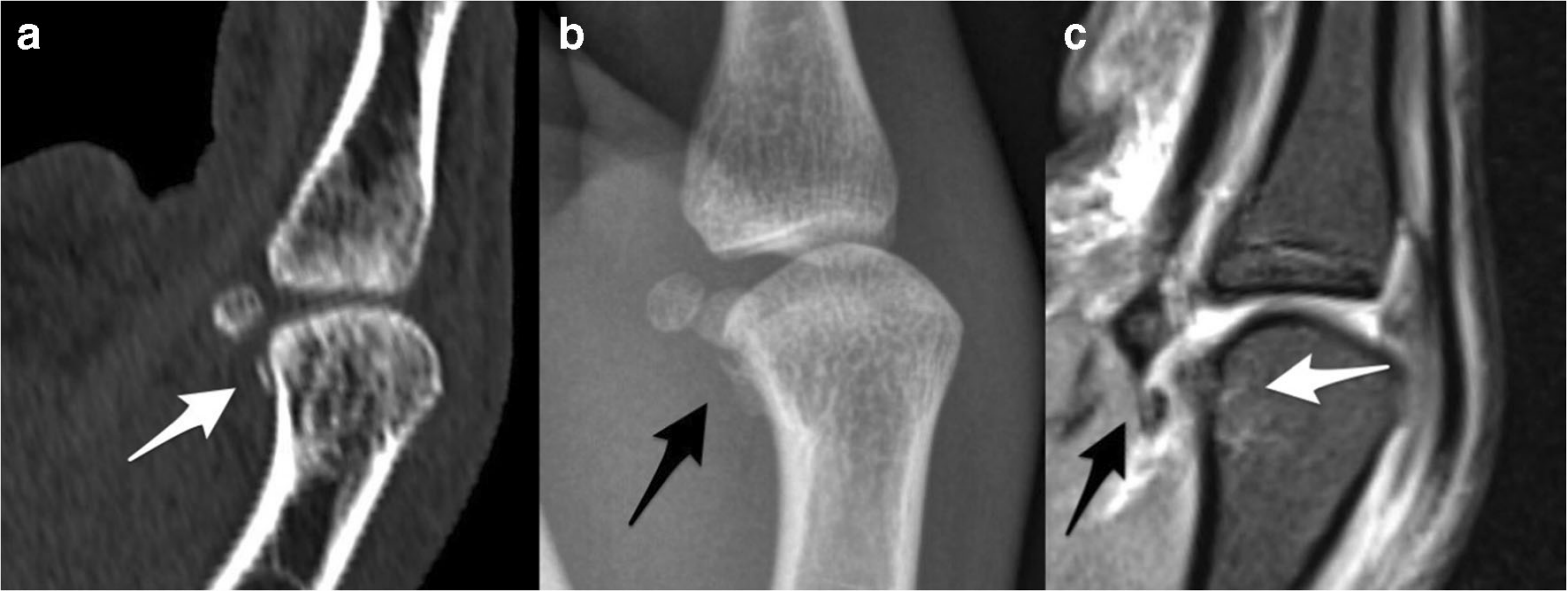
Sagittal reformatted computed tomographic image (a), lateral radiograph (b), and sagittal intermediate-weighted fat-saturated image (c) demonstrate a bony avulsion at the proximal insertion of the checkrein ligament (white arrow in a, black arrows in b and c). Note the subtle bone marrow edema pattern (white arrow in c) at the volar aspect of the metacarpal head adjacent to the bony avulsion
